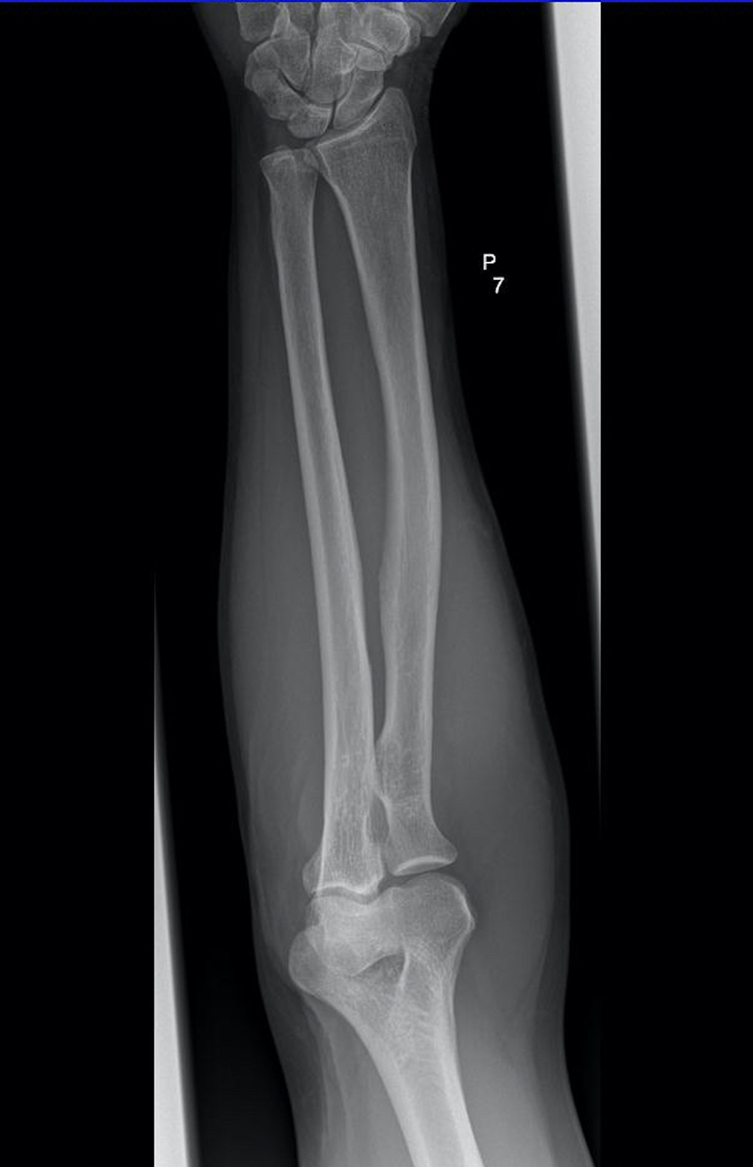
Plain AP radiograph of the forearm and elbow showed no obvious soft tissue mass in the region of extensor digitorum muscle or evidence of any bone destruction.
